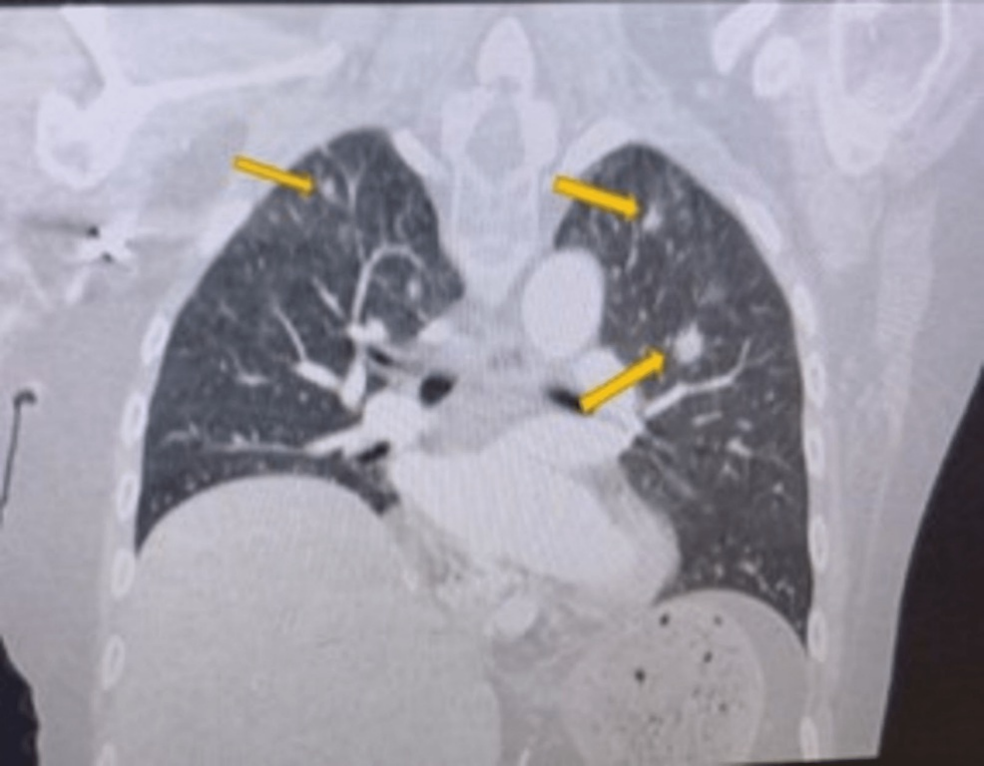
Contrast-enhanced chest CT showing bilateral noncalcified lung nodules.
